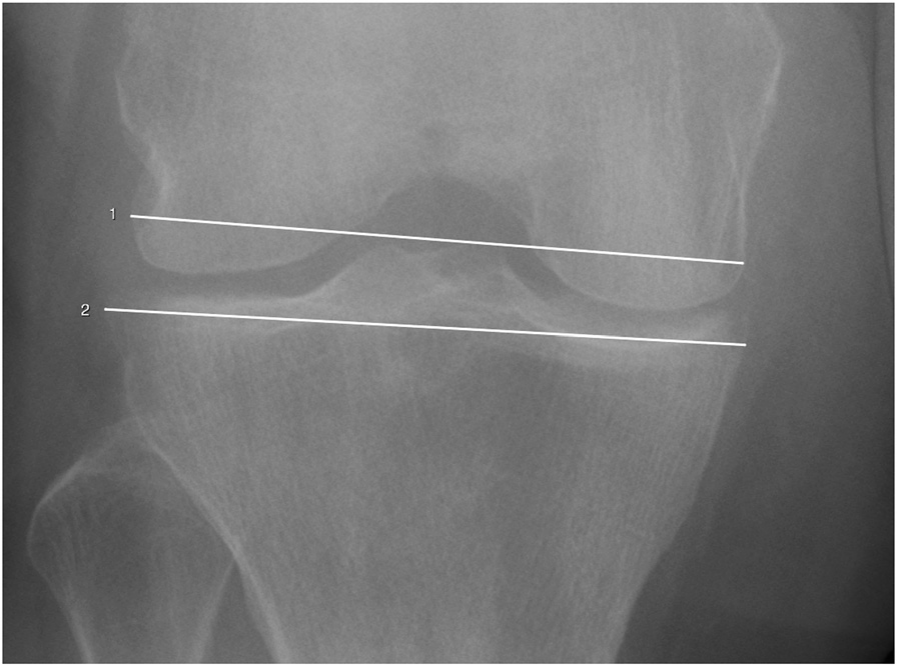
Method of measurements on plain radiograph anteroposterior (right knee – unaffected side): line 1 was drawn between the cortices of the medial and lateral condyle near the articular surface, line 2 was drawn from medial to lateral rim of the proximal tibia (without osteophytes). Tibial widening was given as the quotient of these two parameters and expressed as a percentage of the femoral width
